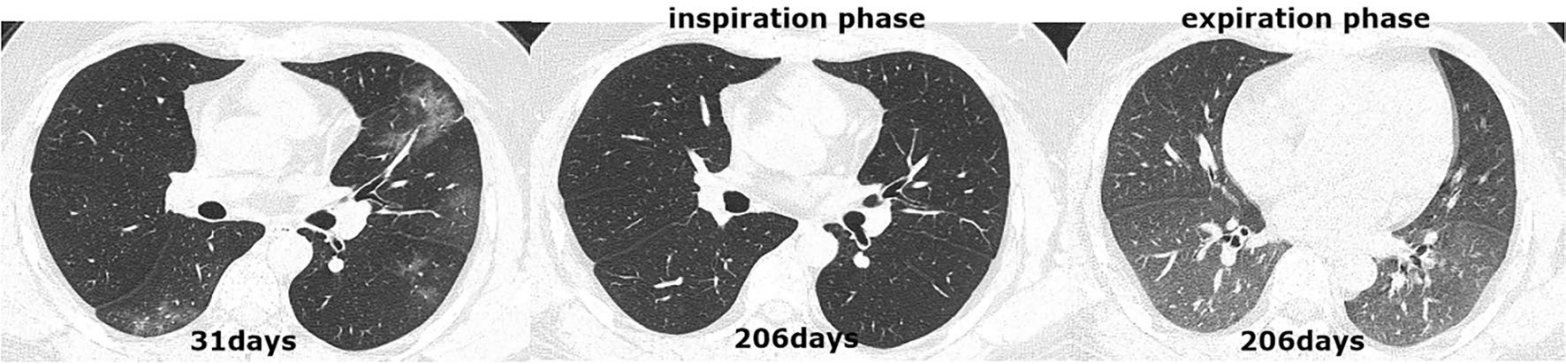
CT scan series in a 65-year-old COVID-19 patient with abnormal DL_CO_ (61.0%) at the six-month follow-up. (a) Transverse CT scan obtained 31 days after the onset of symptoms showed multiple consolidations with ground-glass opacities bilaterally. (b) Scan obtained during full inspiration on 206 days showed that previous opacifications were markedly dissipated subpleural, irregular linear opacities. (c) Scan obtained during expiration at 200 days showed substantial air trapping.
